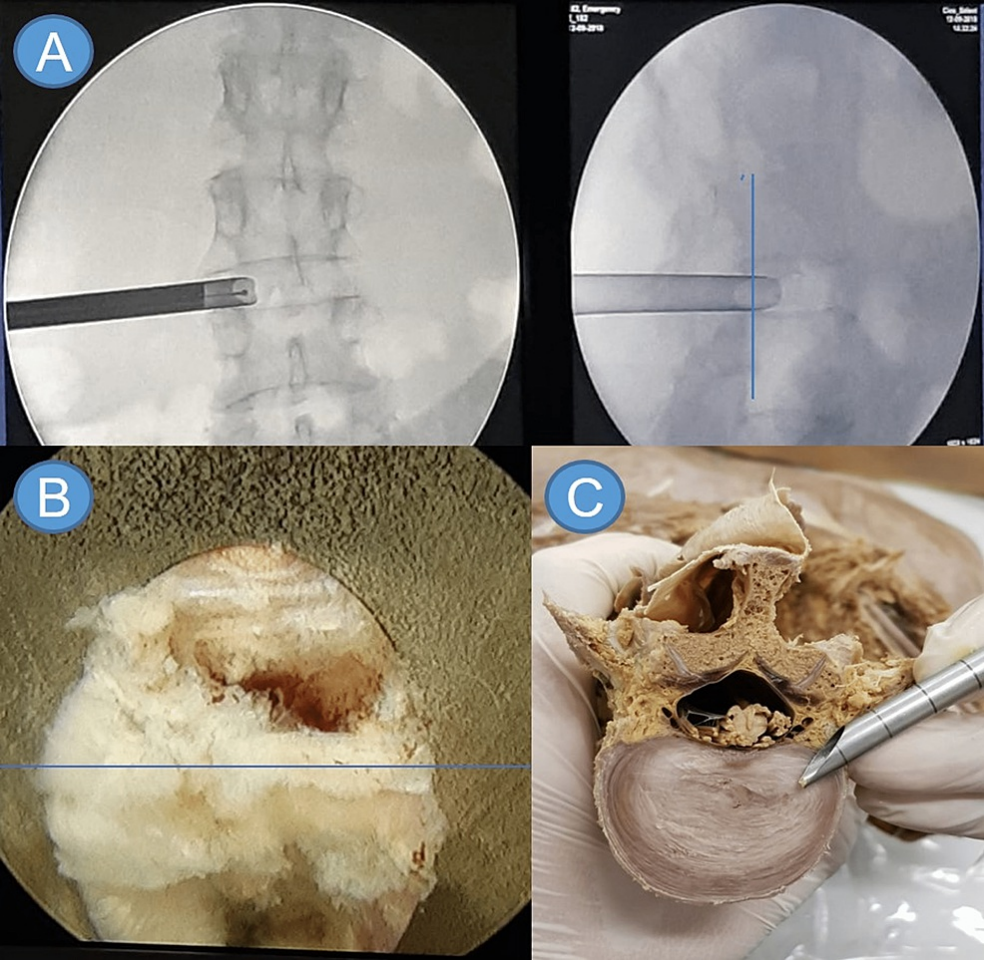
(A) Intraoperative imaging of the endoscope’s position (from left to right: anteroposterior and profile lumbar spine radiography); (B) endoscopic view of “half and half”; (C) representation of the endoscope’s position using an anatomical model (L2). Source: author
